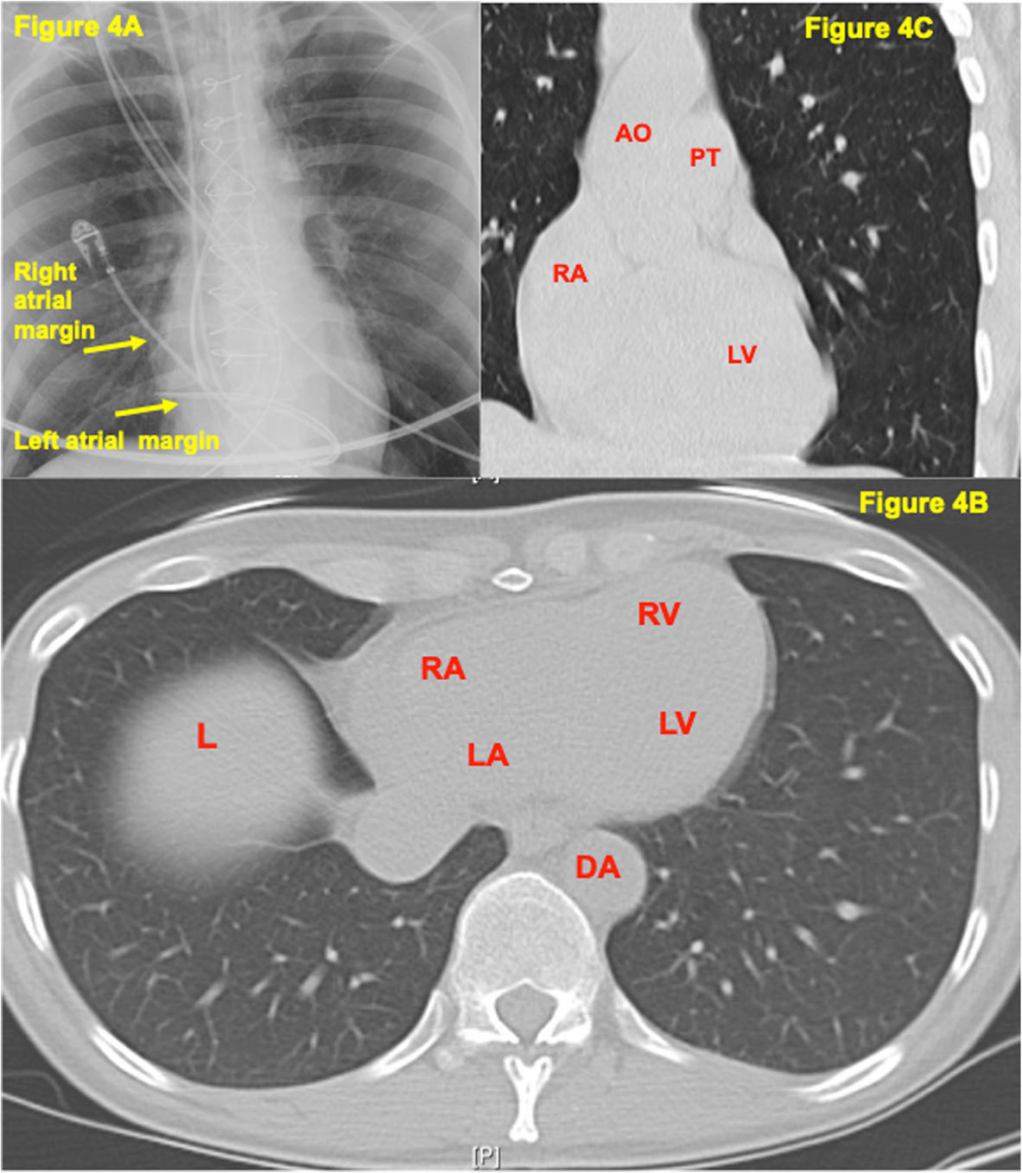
a: Peri-operative chest xray shows “double density” behind the right heart border, a common indication of left atrial enlargement. b: Pre-operative CT chest without contrast, axial view showing limited anatomical detail. Intracardiac mass not discernable. Abbreviations: RA-right atrium, RV-right ventricle, LA-left atrium, LV-left ventricle, DA-descending aorta, L-liver. c: Pre-operative, CT chest without contrast, coronal view showing limited mediastinal detail, left atrium myxoma is not apparent or noticeable. Abbreviations: RA-right atrium, AO-Ascending aorta, PT-pulmonary trunk, LV-left ventricle
